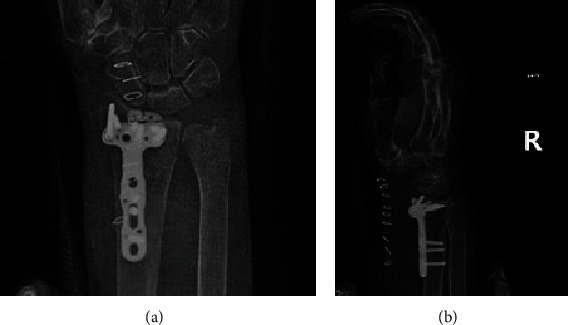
Postoperative plain radiographs of the right wrist showing on the (a) AP and (b) lateral views reduction of the distal radius fracture using a distal volar plate and nine screws.
